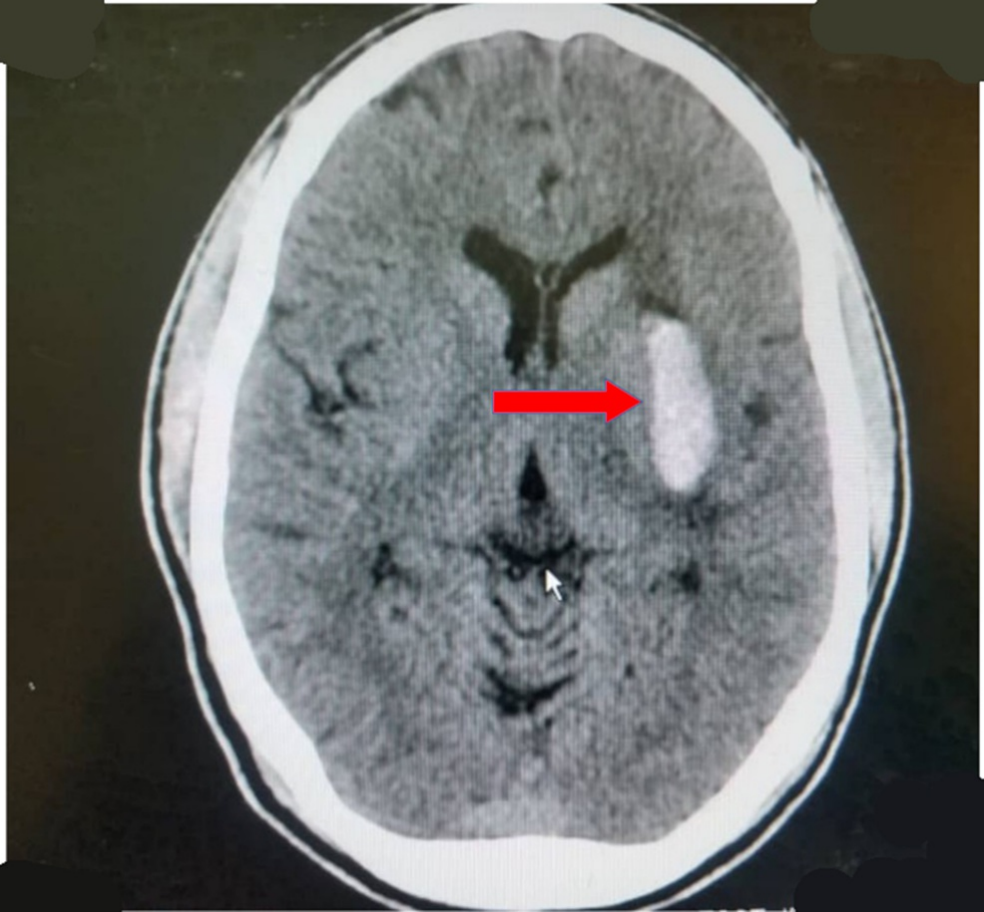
An axial non-enhanced CT scan of the brain of a 49-year-old woman, a known hypertensive who presented with a sudden onset of right hemiparesis, showing an area of hyperdensity at the left basal ganglia (red arrow) with minimal perilesional edema in keeping with acute left basal ganglia hemorrhage.
